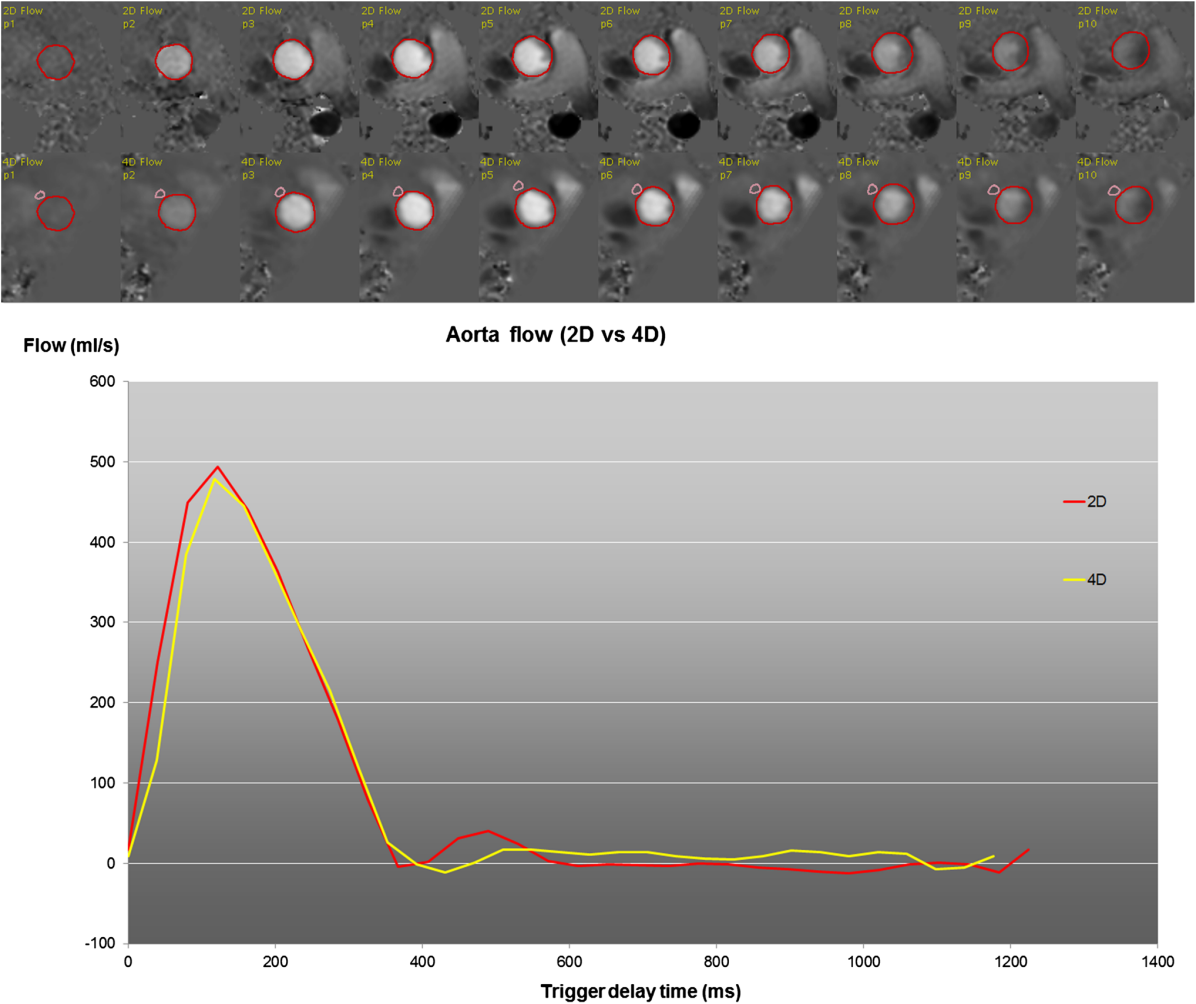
Example of aortic flow curves of a healthy subject derived from conventional 2D phase-contrast MRI (2D Flow) and from a reformatted 4D Flow acquisition. The 4D Flow acquisition was reformatted into a through-plane encoded view, identical to the corresponding 2D Flow acquisition. The top two rows depict the systolic through-plane velocity-encoded images (the first 10 frames out of 30) for 2D Flow and 4D Flow MRI, respectively. Contours are defined around the aortic lumen (shown in *red*). In the 4D Flow-derived images, an additional contour is defined in a region of tissue adjacent to the aorta for velocity offset correction. A good agreement is observed between the two flow curves. The stroke volume derived from 2D Flow is 105 ml and from 4D Flow is 103 ml (Color figure online)
